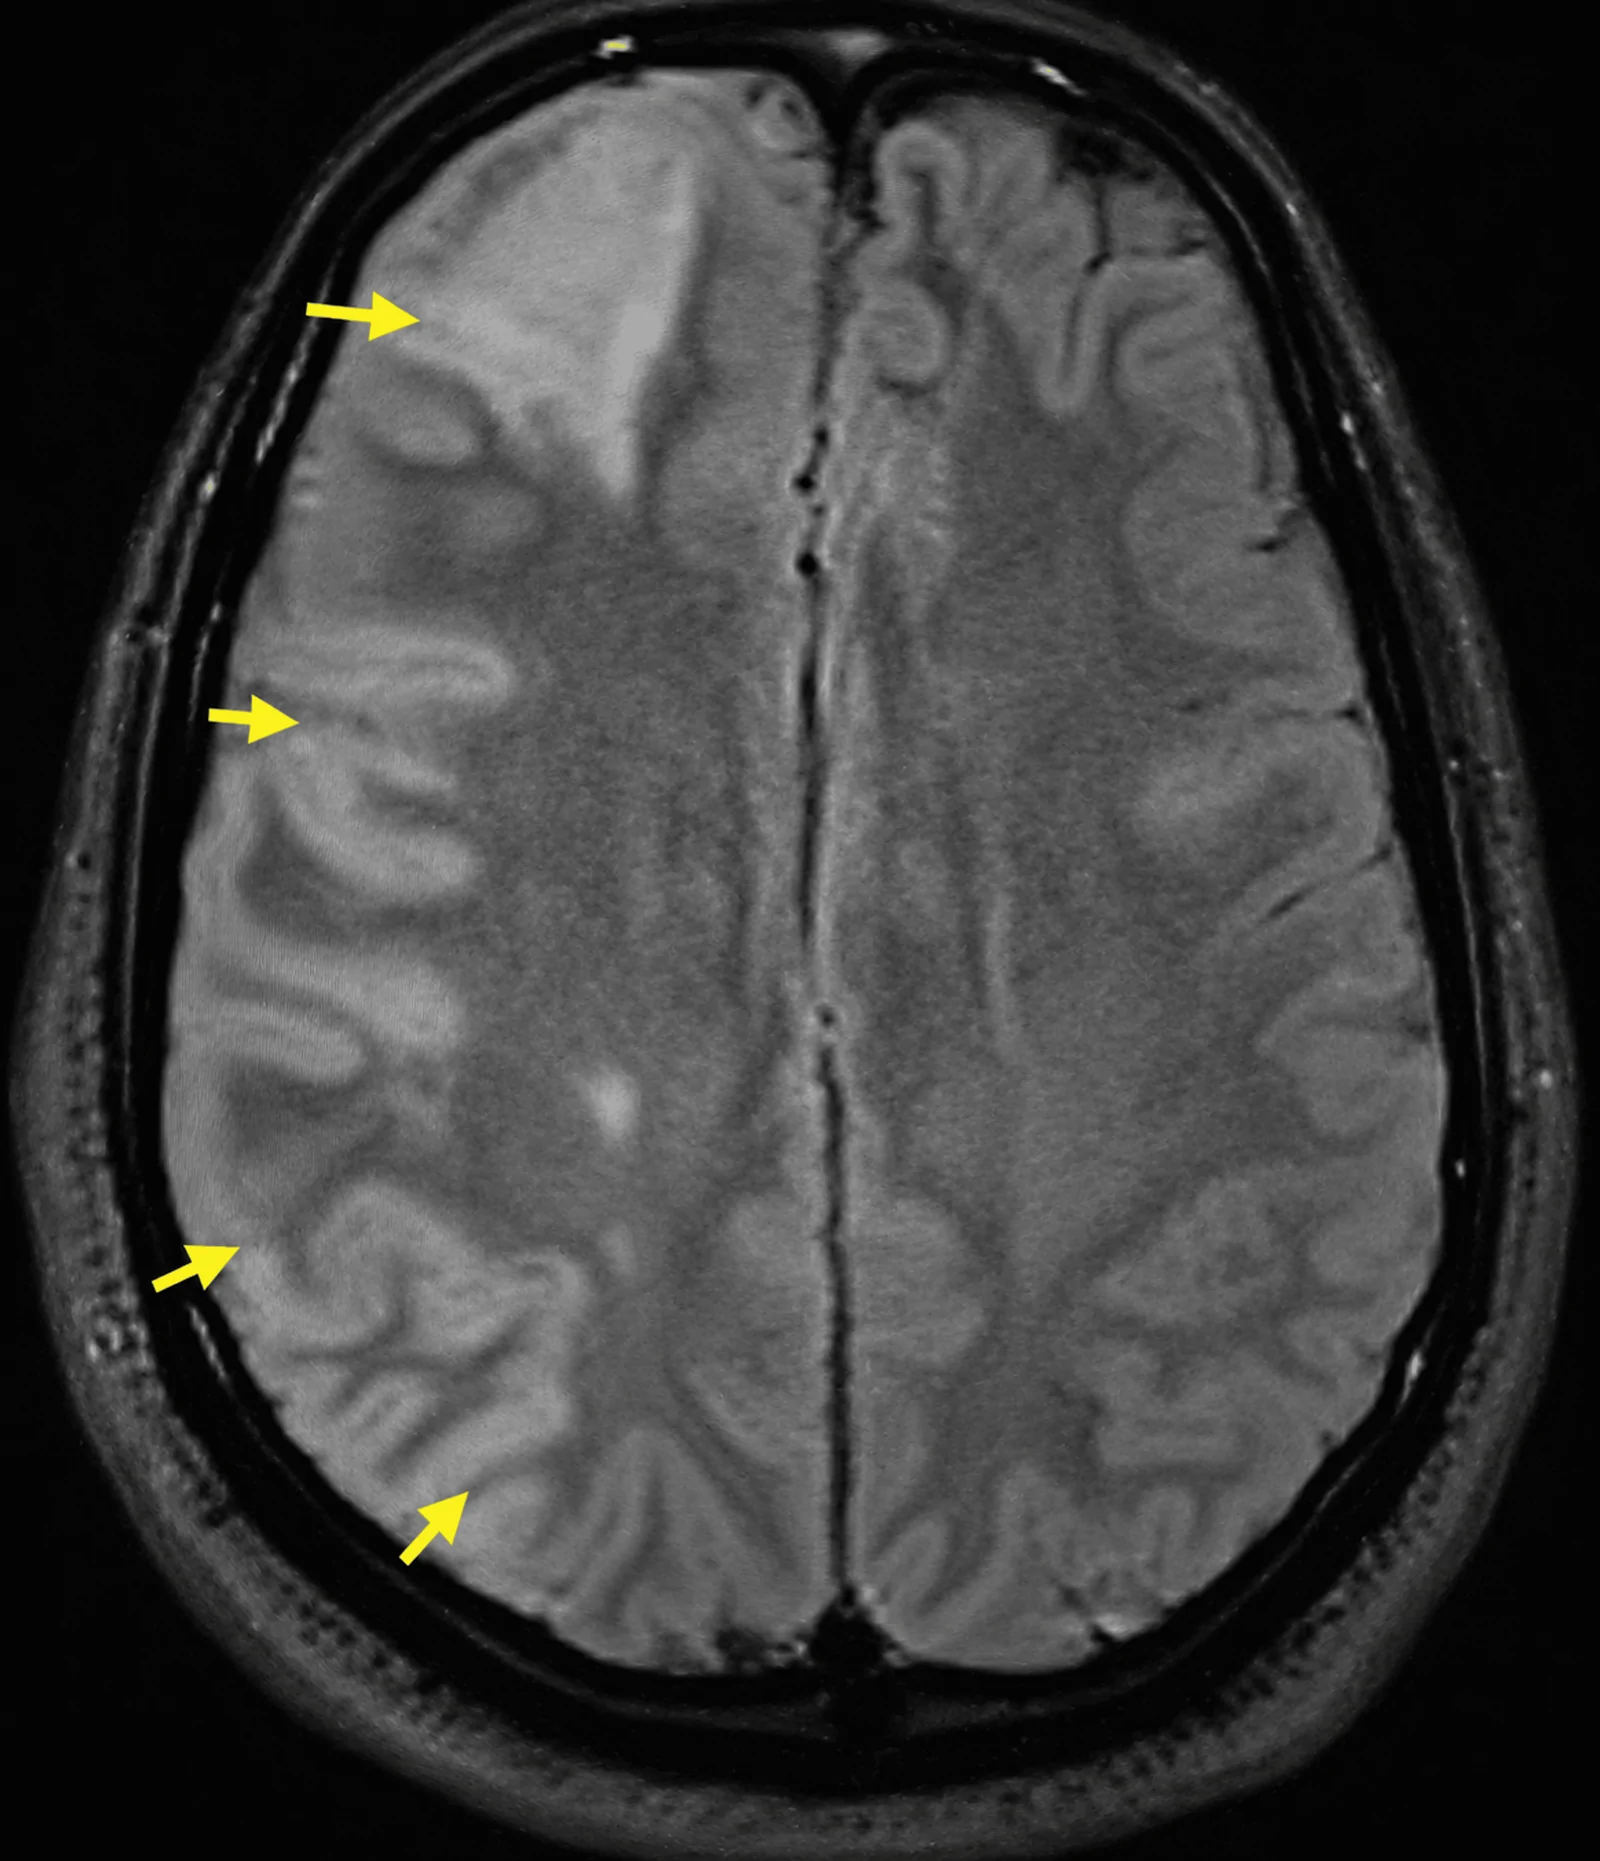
Axial FLAIR MRI showing right frontoparietal cortical and subcortical hyperintensities consistent with infectious meningoencephalitis (yellow arrows) The FLAIR hyperintense abnormalities involve the right frontoparietal cortex and adjacent subcortical white matter (yellow arrows), reflecting parenchymal involvement in the setting of infectious meningoencephalitis. FLAIR: fluid-attenuated inversion recovery
